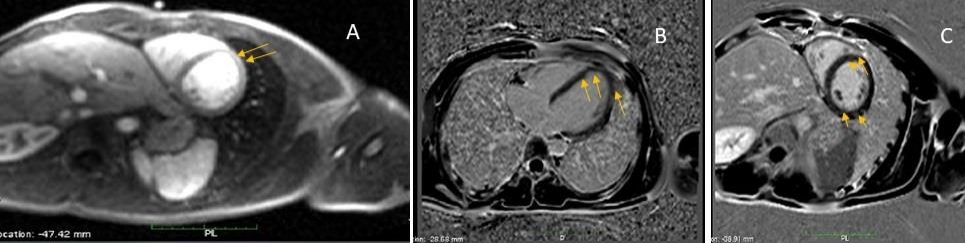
A) cardiac magnetic resonance (CMR) showed increased signal intensity at the mid-lateral wall on T2-weighted images; late enhancement revealed a patchy subepicardial hyperenhancement of the medio-distal segments of the anterior and anterolateral walls (B and C)
